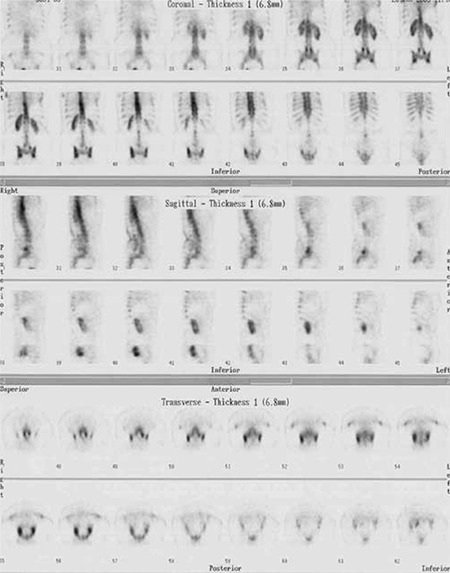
Coronal, sagittal and transaxial slices of SPECT images of the same patient
